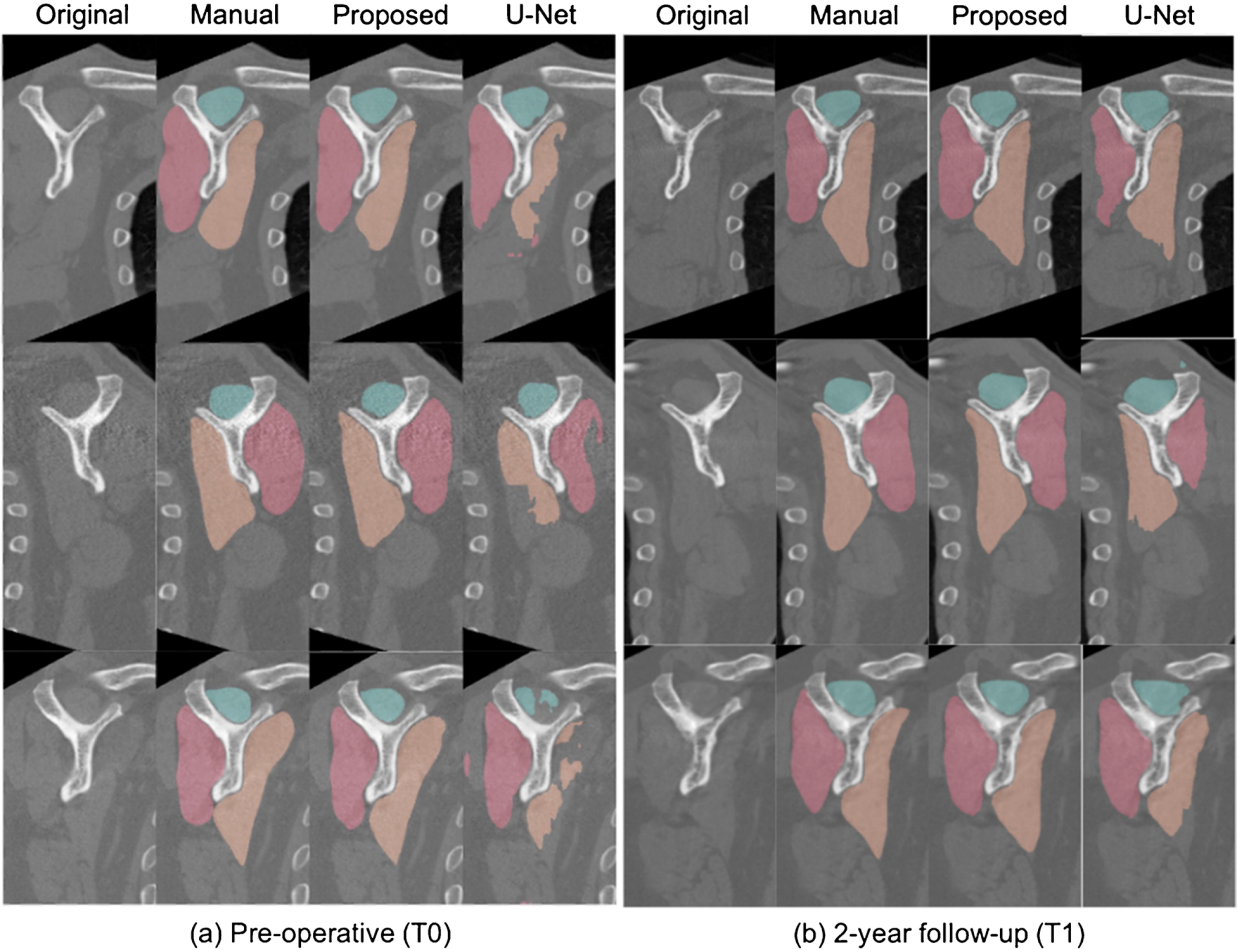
Examples of rotator cuff muscle segmentation on CT slices collected at a pre-operative and b 2-year follow-up from three test cases with different segmentation methods. Supraspinatus is depicted in green; infraspinatus/teres minor is in red; and subscapularis is in orange
